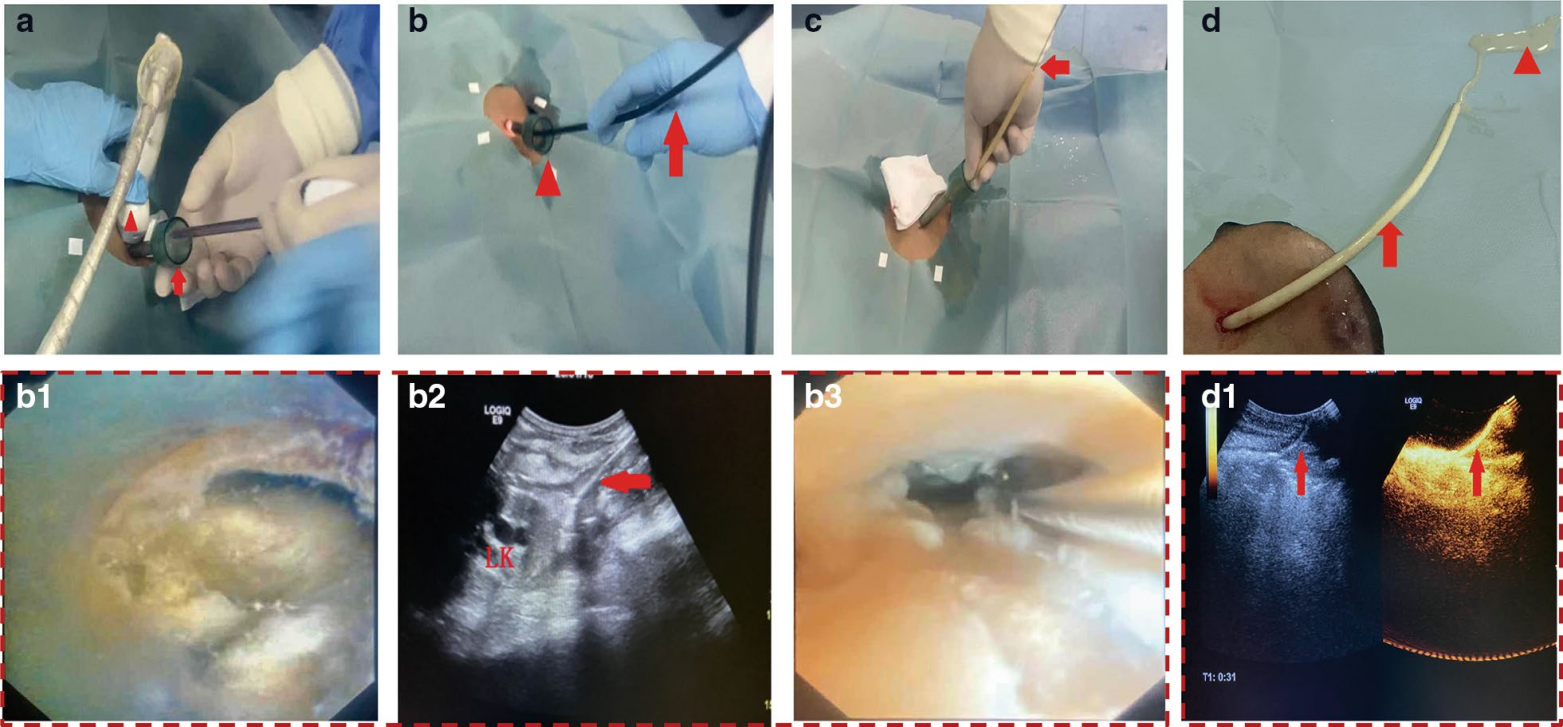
Images of modified percutaneous catheter drainage under the triple guidance of choledochoscopy, ultrasonography, and computed tomography. a After puncture by the trocar (red arrow), the core is withdrawn to establish a channel for the choledochoscope. b The cholangioscope (red arrow) is introduced through the trocar (red triangle), and the condition of the inner walled-off necrosis (WON) is observed by the choledochoscope (b1) and ultrasonography (b2, red arrow indicates the choledochoscope) in real time, followed by introduction of a guide wire to target the lowest part of the WON (b3). c A multi-side-hole catheter (red arrows, 16 Fr) is inserted along the guidewire to fully drain the WON, and abscess accumulation is observed by contrast-enhanced ultrasonography. d The liquefied necrotic tissue (red triangle) is successfully drained using the catheter (red arrow) and verified by contrast-enhanced ultrasonography (d1)
